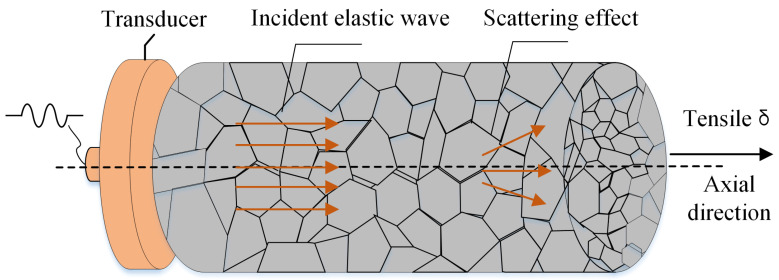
Ultrasonic wave propagation in bolt under the axial stress.
